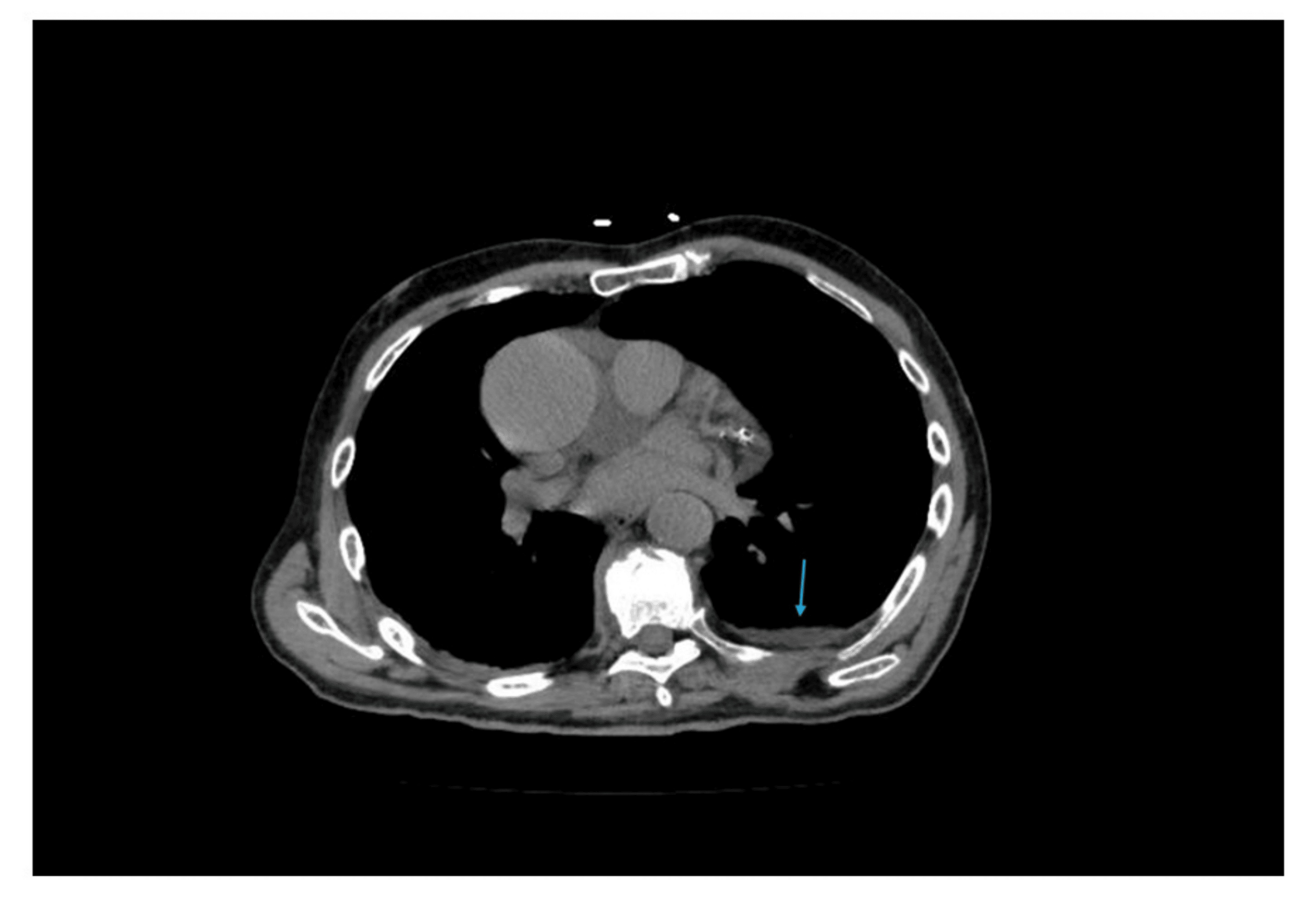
Chest computed tomography (CT) demonstrating left pleural effusion (light blue arrow).
